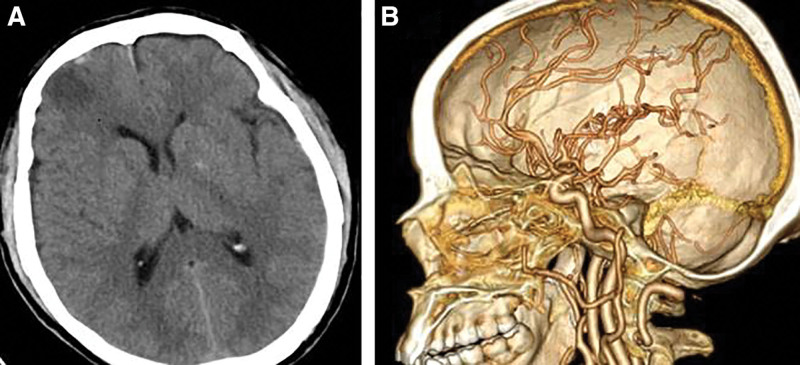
(A) Computed tomography scan of the bilateral frontal and temporal lobe brain contusion with a small hematoma, left temporal subdural hematoma, and left temporal parietal bone linear fracture. (B) Computed tomographic angiography showing no right anterior cerebral artery aneurysms.
